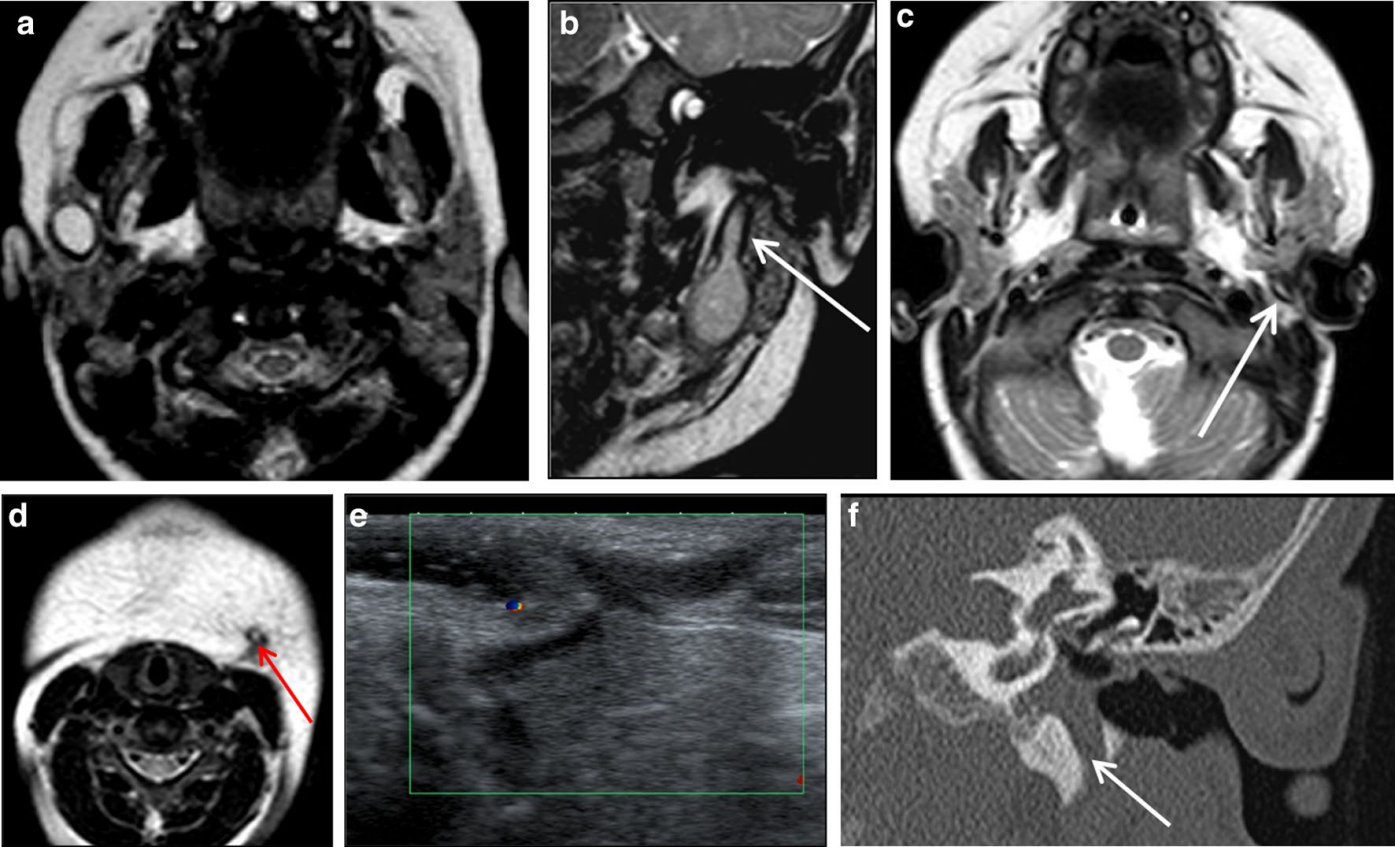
First branchial-cleft cyst and fistulas. Right isolated intraparotid cyst on axial *T*_2_-weighted image (a). Type II anomaly, with an intraparotid cyst and 2 fistulas, the upper fistula running from the cyst to the external auditory canal [white arrow] and the lower running from the cyst to the skin, in Pochet’s triangle [red arrow] (b, c and d). Isolated fistula running from the parotid area to the skin (e). Congenital defect of the floor of the left external auditory canal (tympanal bone) on frontal and axial CT images (f and g)
